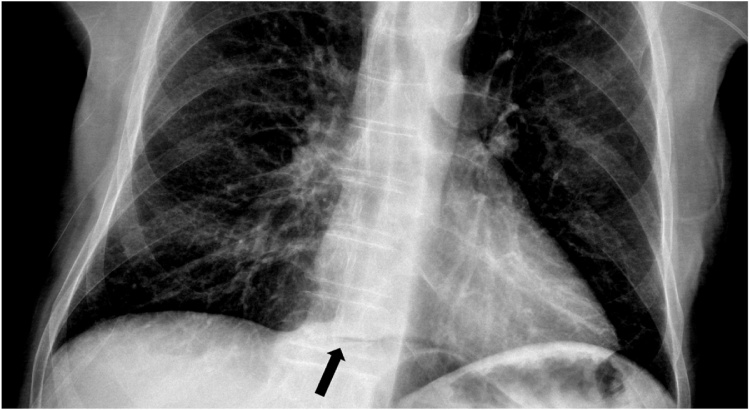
Chest X-ray showing subdiaphragmatic free air along the midline.
